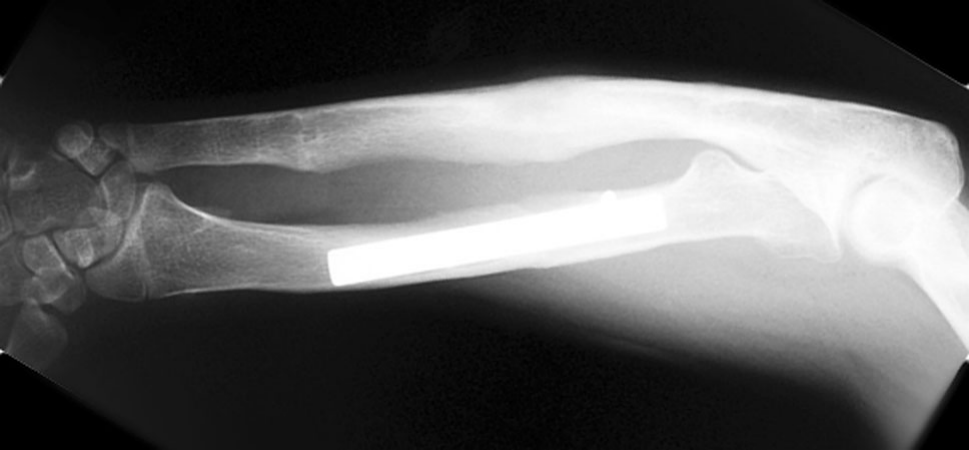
X-ray after removal of the external fixator, fully corrected deformity with excellent regenerate (note the 0.5 over-lengthening to avoid complications of recurrence and to improve the function
